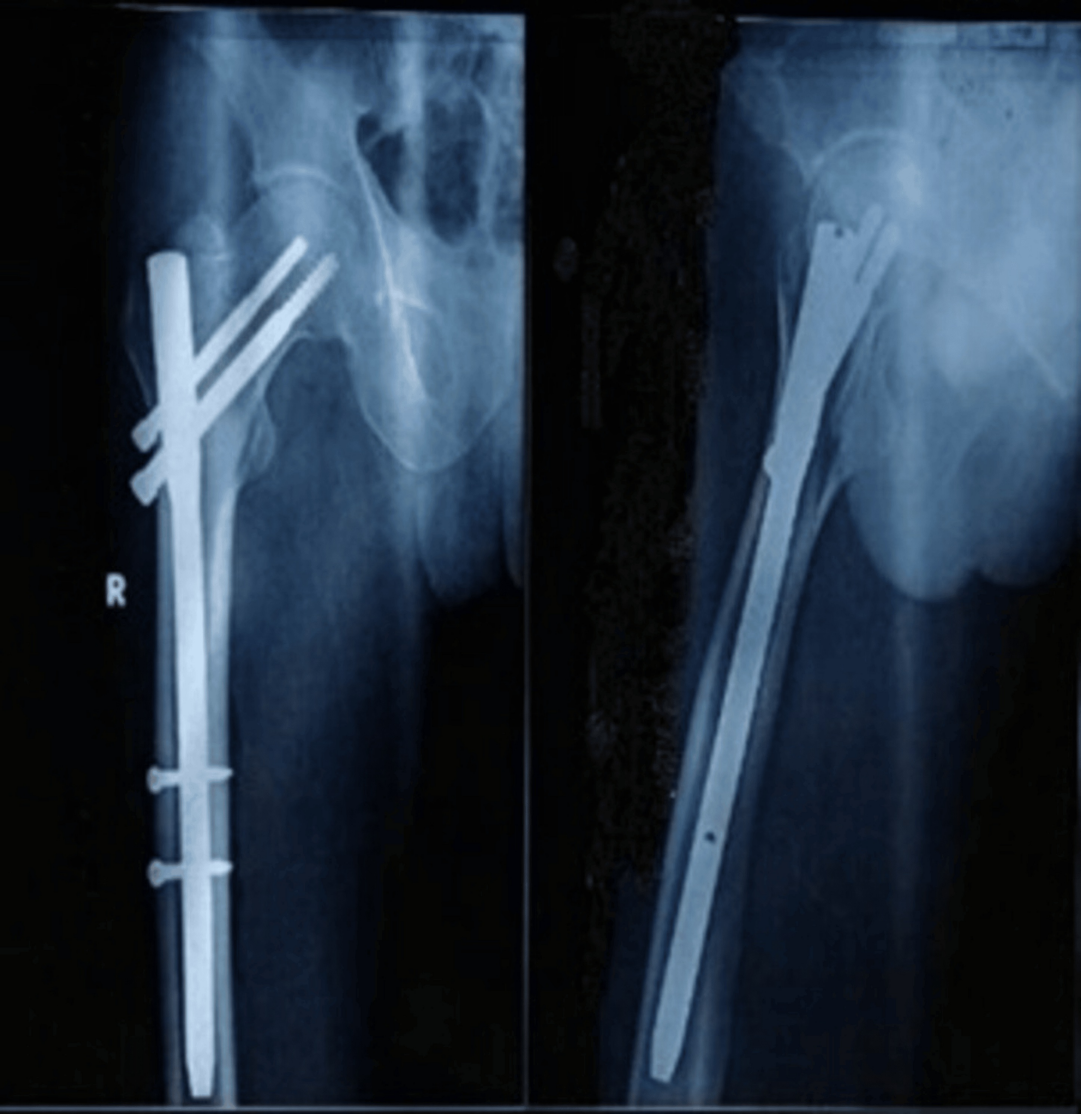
Eight-month follow-up radiograph of the same patient The patient, whose radiograph is shown in Figure 4, underwent AP and lateral radiographic imaging of the right hip joint and femur six months postoperatively, showing radiological union of the intertrochanteric fracture. AP: anteroposterior.
